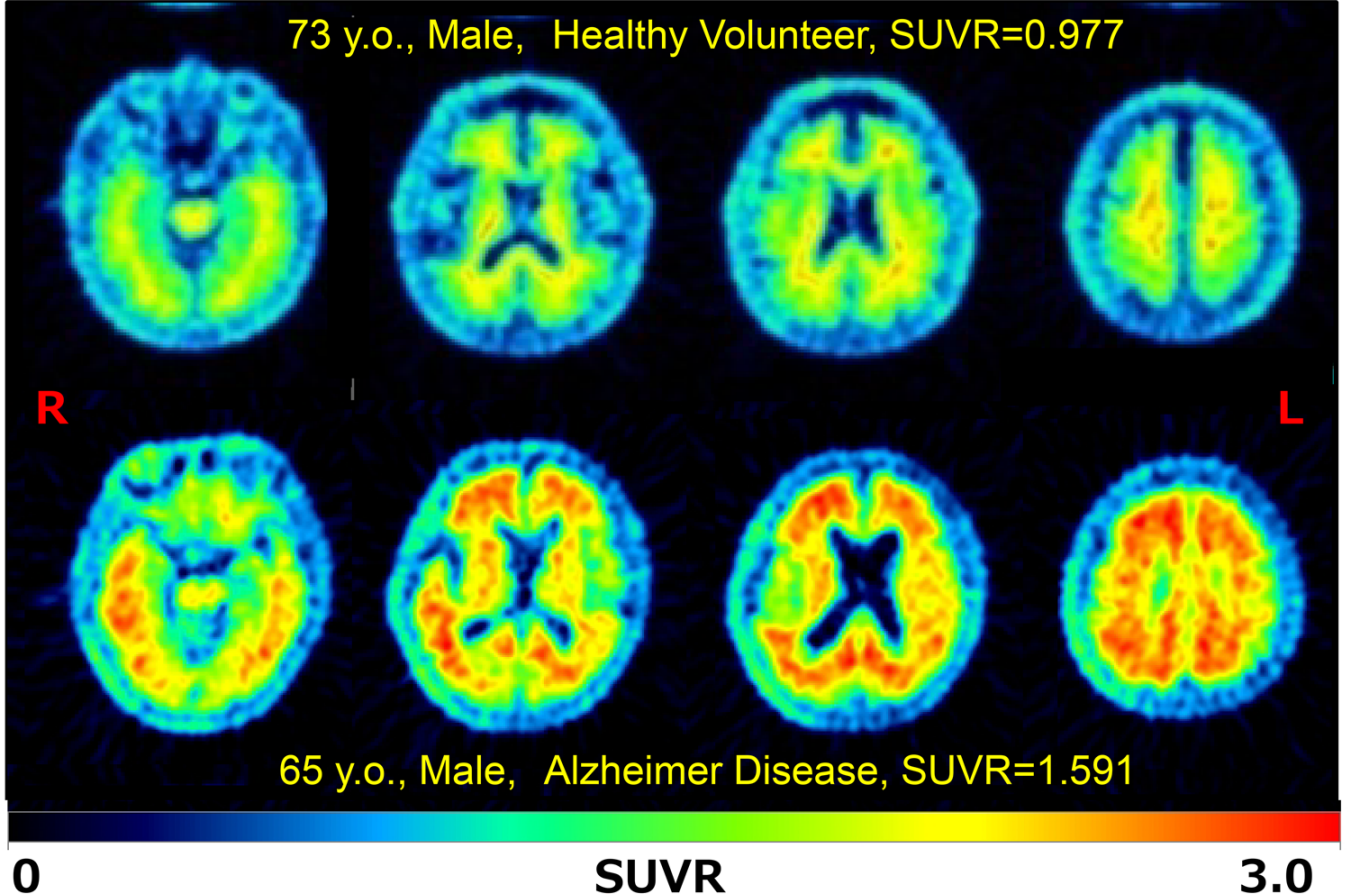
Representative brain axial PET images of ^18^F-FPYBF-2 PET in a healthy volunteer and a patient with Alzheimer disease. The upper half showed a typical case of healthy 73 years old male volunteer, who has MMSE: 28 with Mean Cortical Index of SUVR as 0.977. The lower half showed a typical case of patient with Alzheimer disease, 65 years old male, who has MMSE: 14 with Mean Cortical Index of SUVR as 1.591. Prominent accumulation of ^18^F-FPYBF-2 was observed in gray matter of frontotemporal cortex, parietal and occipital cortex and posterior cingulate gyrus
